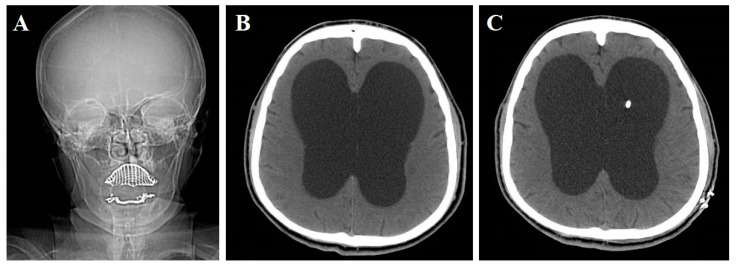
Preoperative skull X-ray showing slight macrocephaly (A), preoperative CT scan (B), and postoperative CT scan of a patient with LOVA that underwent VPS (C).
